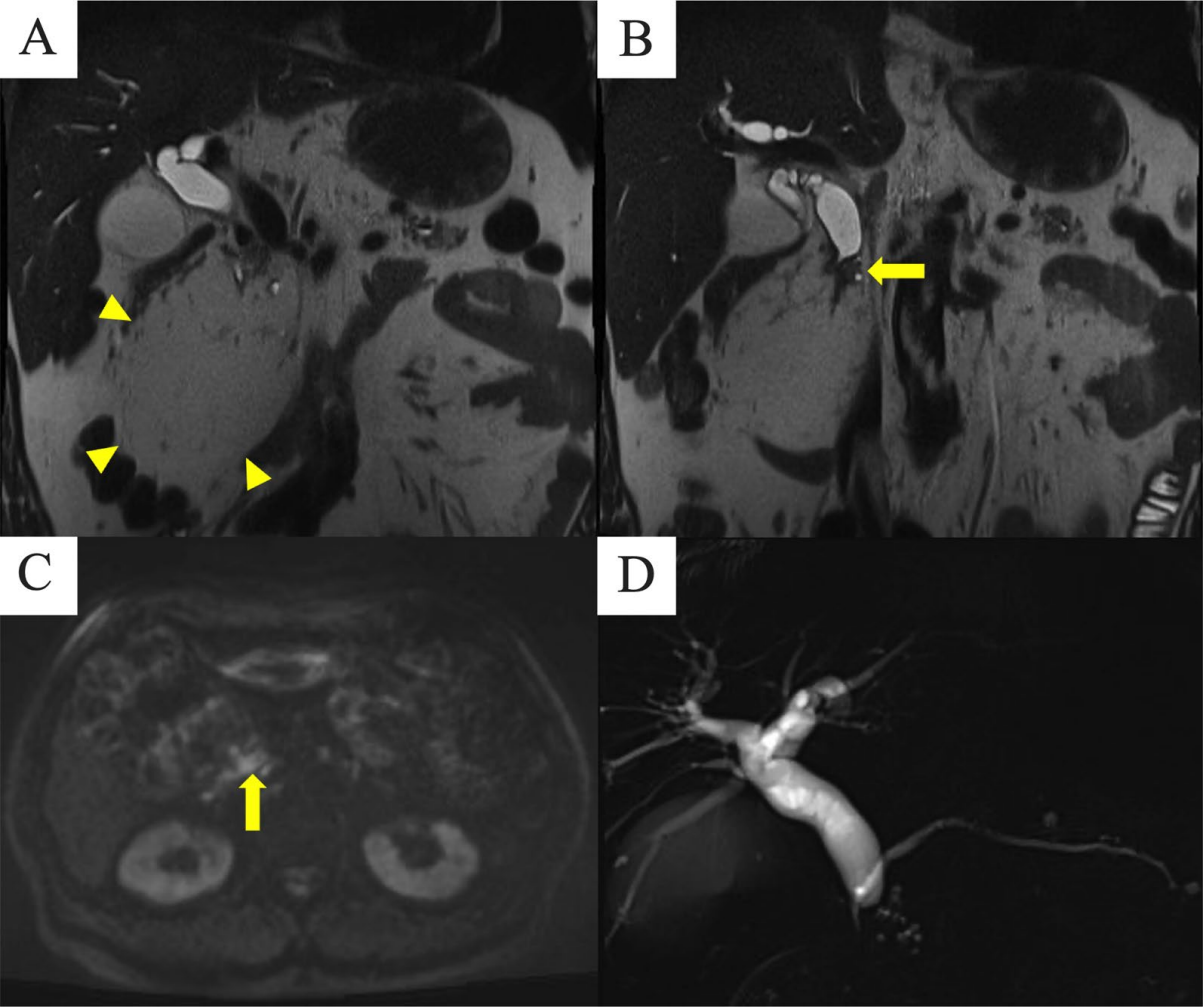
MRI before surgery. A The previously diagnosed 12 cm tumor with homogeneous adipose intensity is visible in the pancreatic head (arrowheads). B A solid T2-low intensity mass appeared at the upper margin of the tumor (arrow), which invaded and obstructed the distal common bile duct. C Diffusion-weighted imaging showed a high-signal intensity mass at the upper margin of the adipose tumor (arrow). D Magnetic resonance cholangiopancreatography showed no stenosis or other specific findings in the main pancreatic duct
